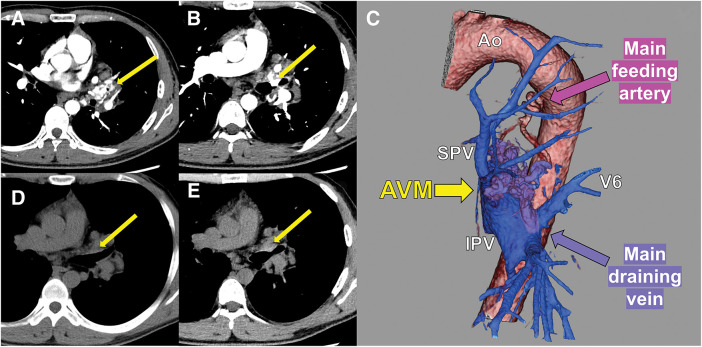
Findings of CT scans. (A and B) Contrast-enhanced CT on admission showing the AVM (yellow arrow) around the left upper lobe bronchus, which protruded into the bronchus. (C) 3D-CT showing the AVM (yellow arrow), which was mainly fed by the left bronchial artery (pink arrow) and drained (purple arrow) into the left IPV. (D) CT performed 4 years before surgery showing no mass (yellow arrow). (E) CT performed 1 year before surgery showing a small endobronchial mass (yellow arrow). Ao: aorta; AVM: arteriovenous malformation; CT: computed tomography; IPV: inferior pulmonary vein; SPV: superior pulmonary vein; 3D-CT: three-dimensional CT
